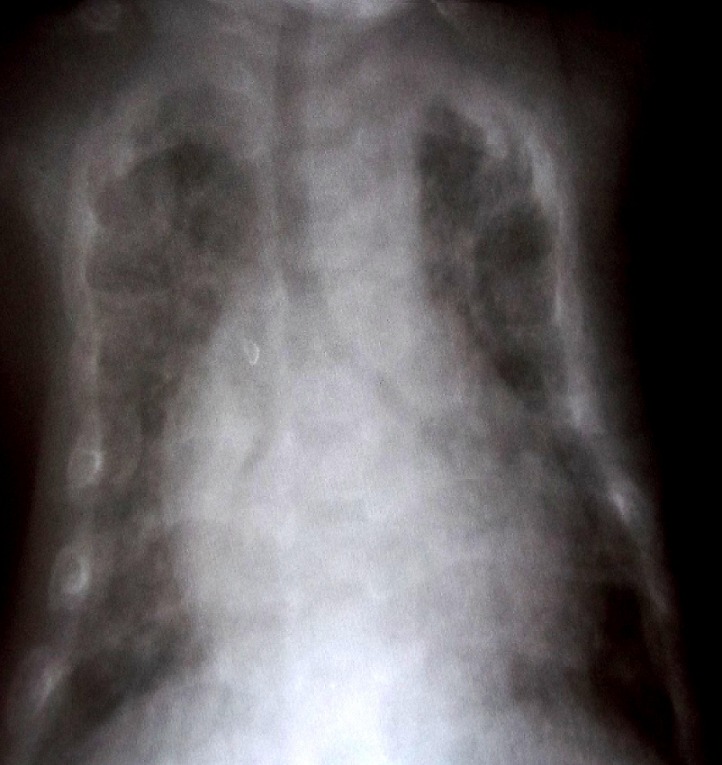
Radiographie du Thorax: Syndrome interstitiel diffus aux deux poumons
